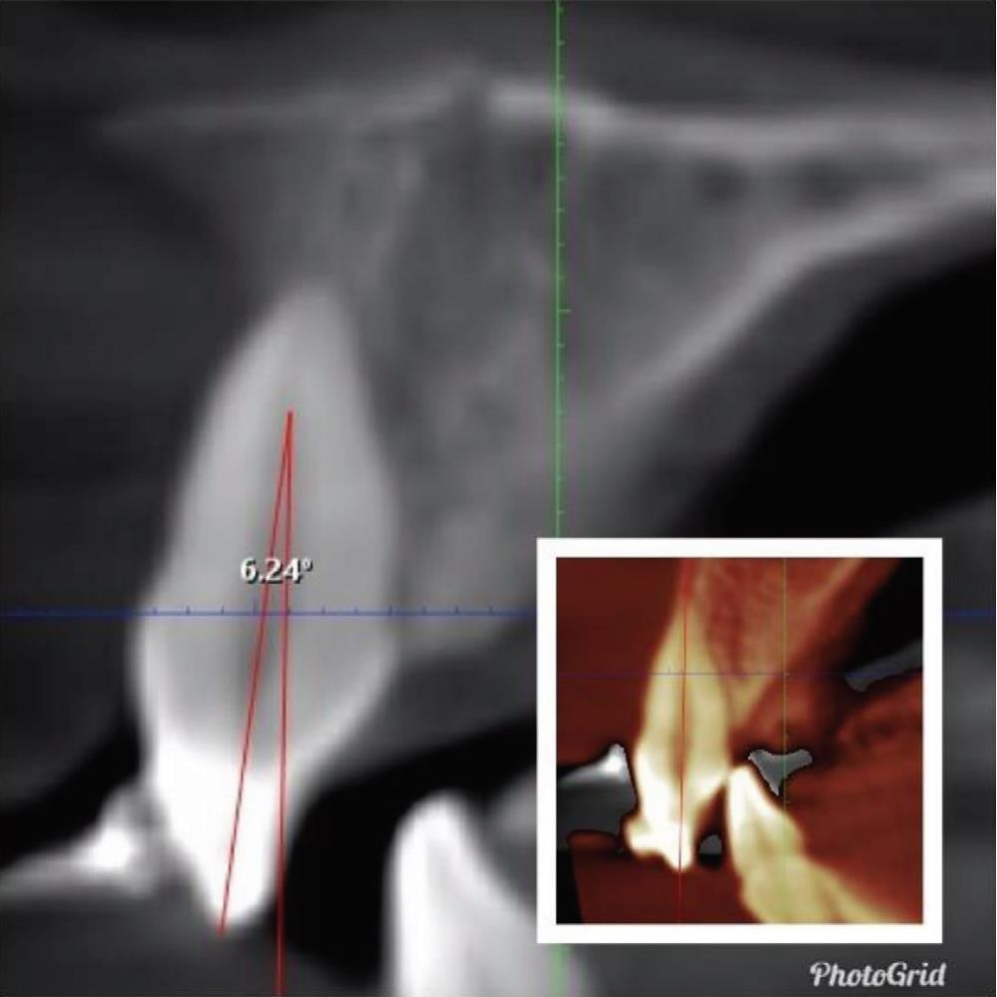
The rate of incisor inclination change with superimposition on the nasal floor in the ULD CBCT image.
